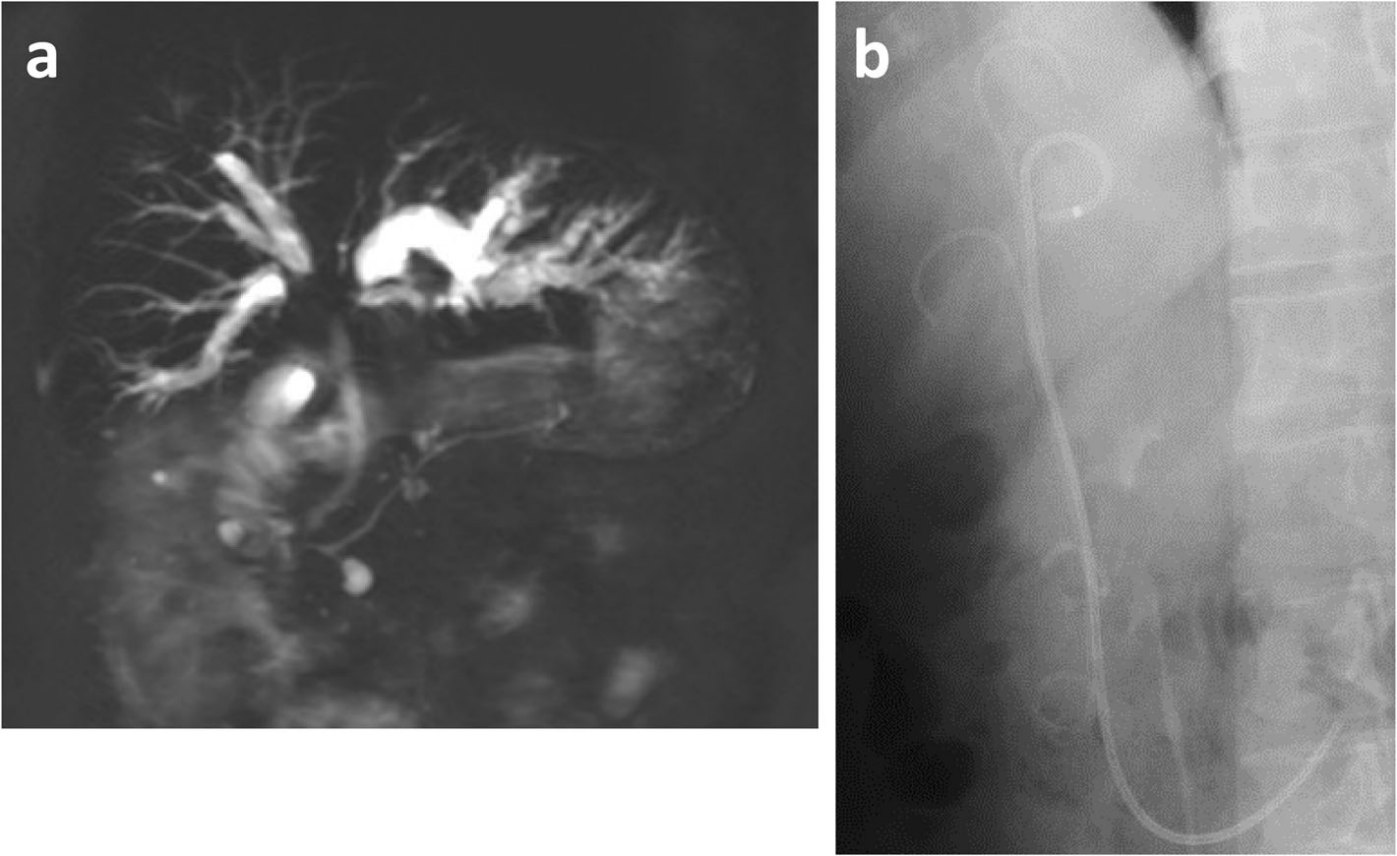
a Magnetic resonance imaging. A metastasis in the biliary hilar region of the liver caused type IV biliary obstruction and bilateral intrahepatic bile duct dilation. b: ERCP imaging. PS had already been placed in the left intrahepatic bile duct (branch of B3 and B2) and right intrahepatic bile duct (B5 and B7). ERCP, endoscopic retrograde cholangiopancreatography; PS, plastic stent
